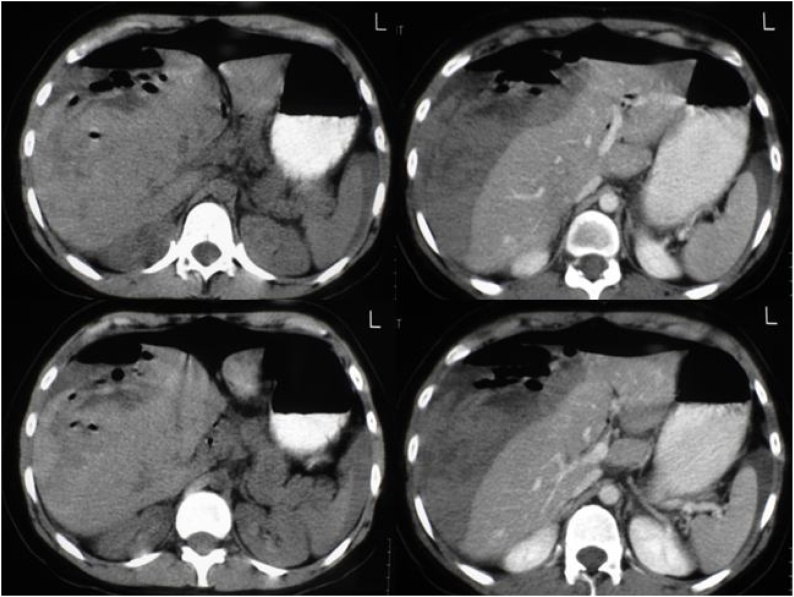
Abdominal CT showing HSH of about 15 cm, affecting liver segments 6, 7 and 8 with permeate air and small amount of perihepatic free liquid.
